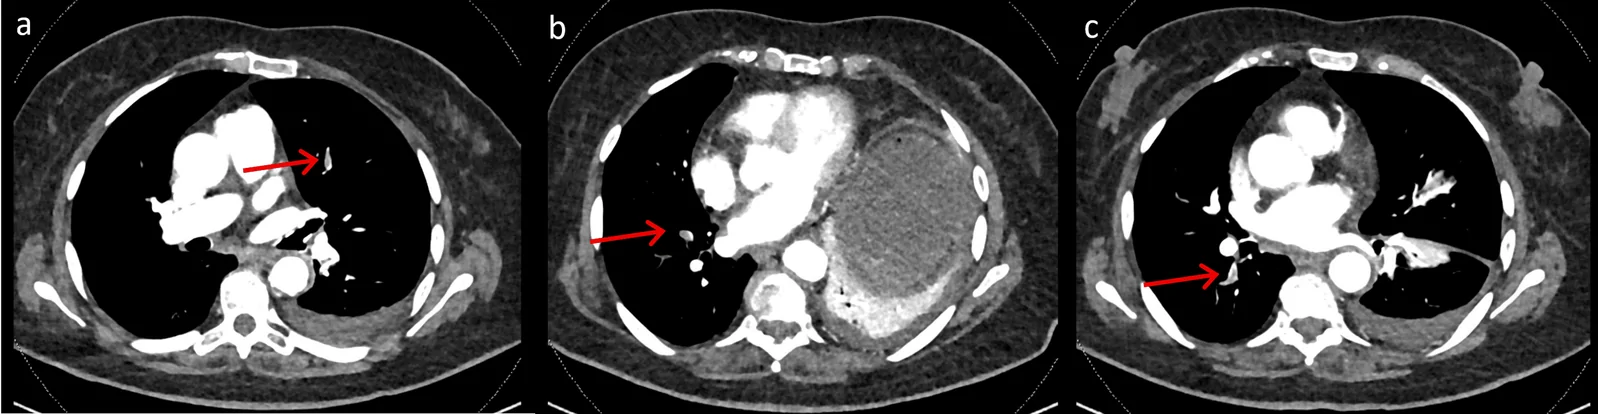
Transversal sections of the thoraco-abdominal CT scan in portal phase; pulmonary embolism indicated by arrows
